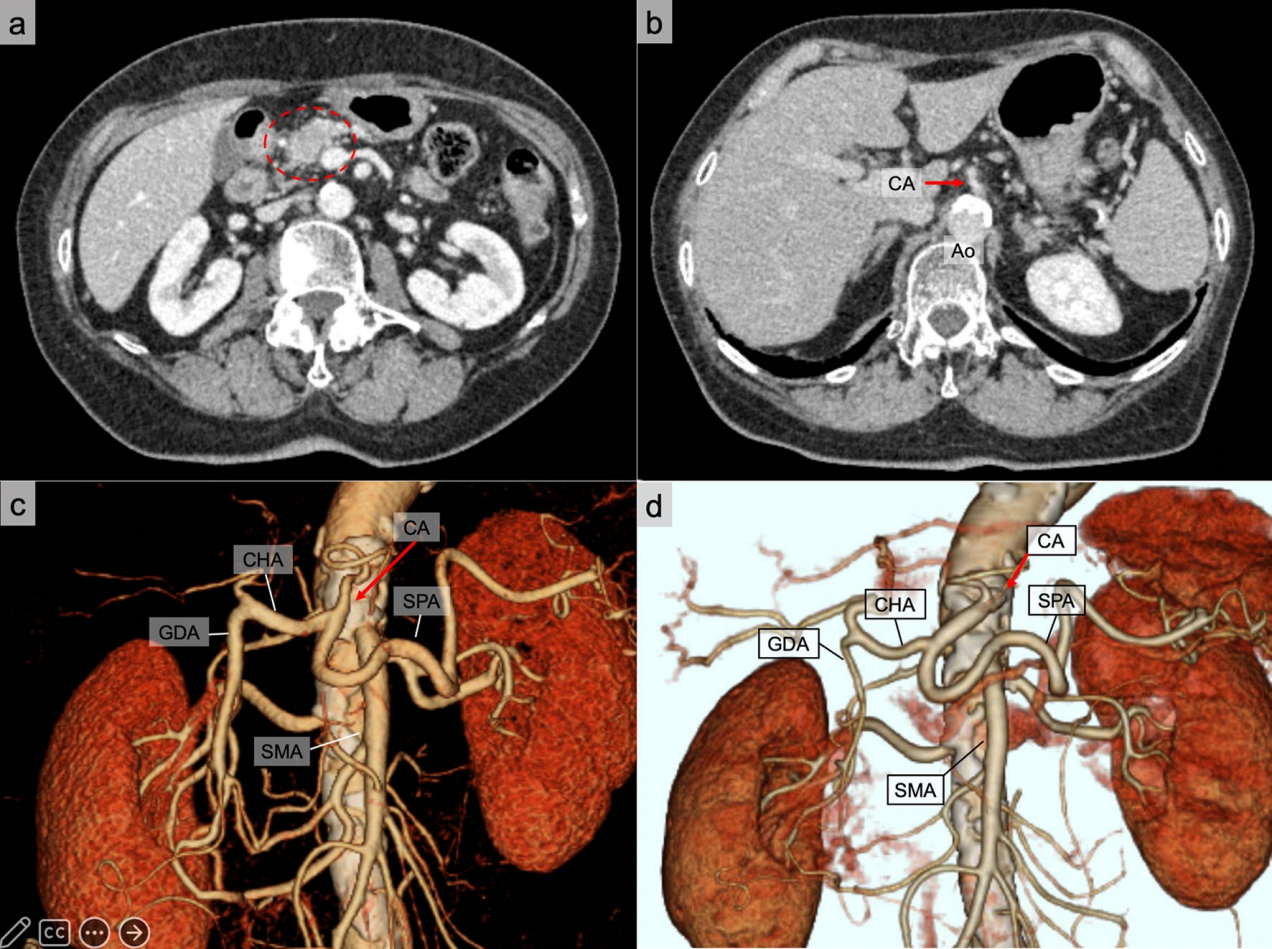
Axial view and three-dimensional reconstruction using contrast-enhanced computed tomography (CT). a An 11 mm × 15 mm low-density mass in the posterior aspect of the pancreatic body with attachment to the superior mesenteric vein (SMV) of less than 180 degrees. b Celiac axis stenosis (CAS) caused by severe calcification. c Well-developed collateral flow from the superior mesenteric artery (SMA) to the gastroduodenal artery (GDA). d After stent placement, the pancreatic arcade became narrower and the PHA became thicker
